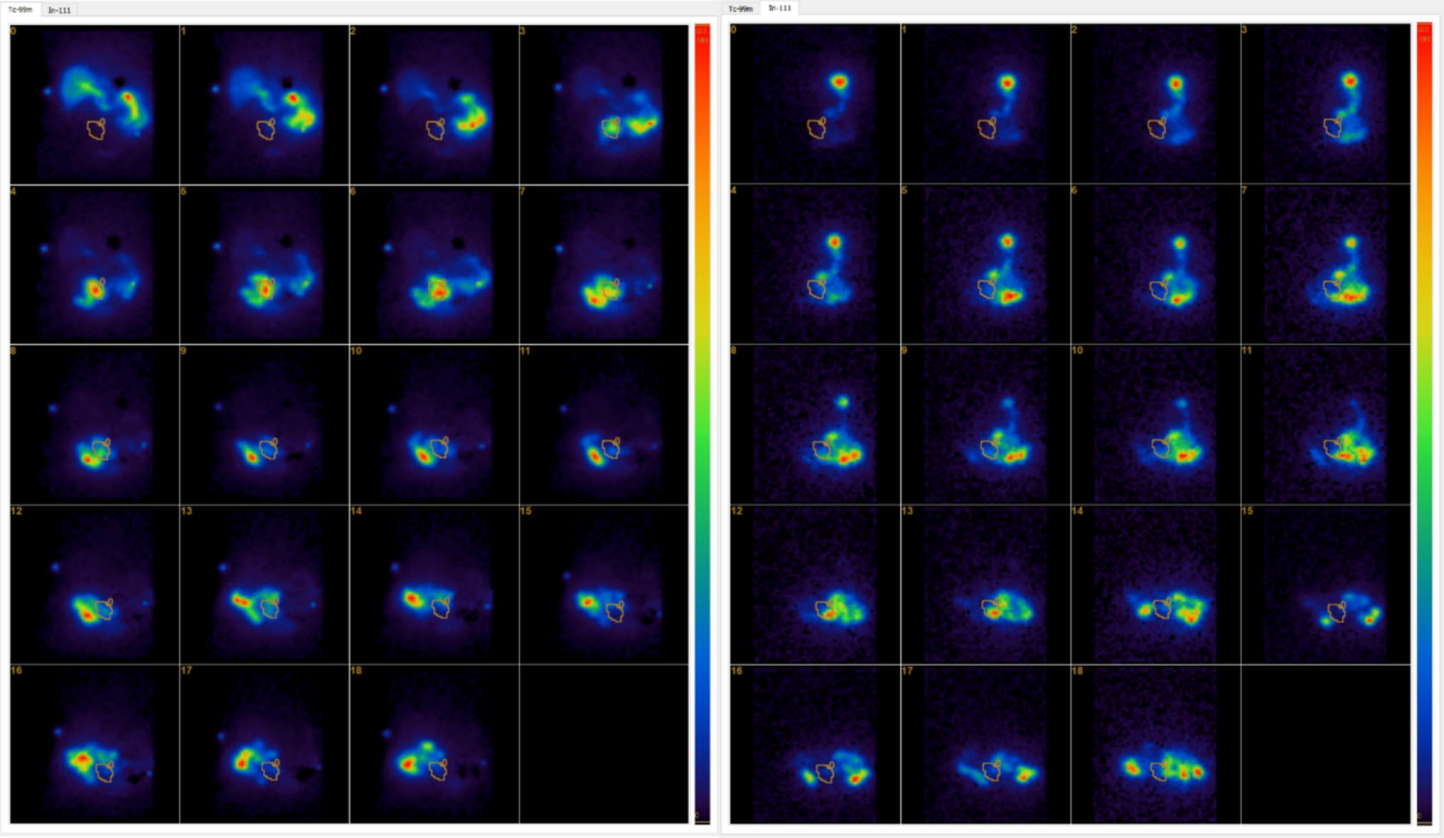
Typical scintigraphy of bile and food transit to the enteroenterostomy (EE). The EE has been drawn with a yellow circle. The color indicates the radioactive uptake ranging from dark blue (low uptake) to red (high uptake). Tc-99 m = 99Tc Bromo-biliaron (bile). In-111 = 111In-DTPA (food)
